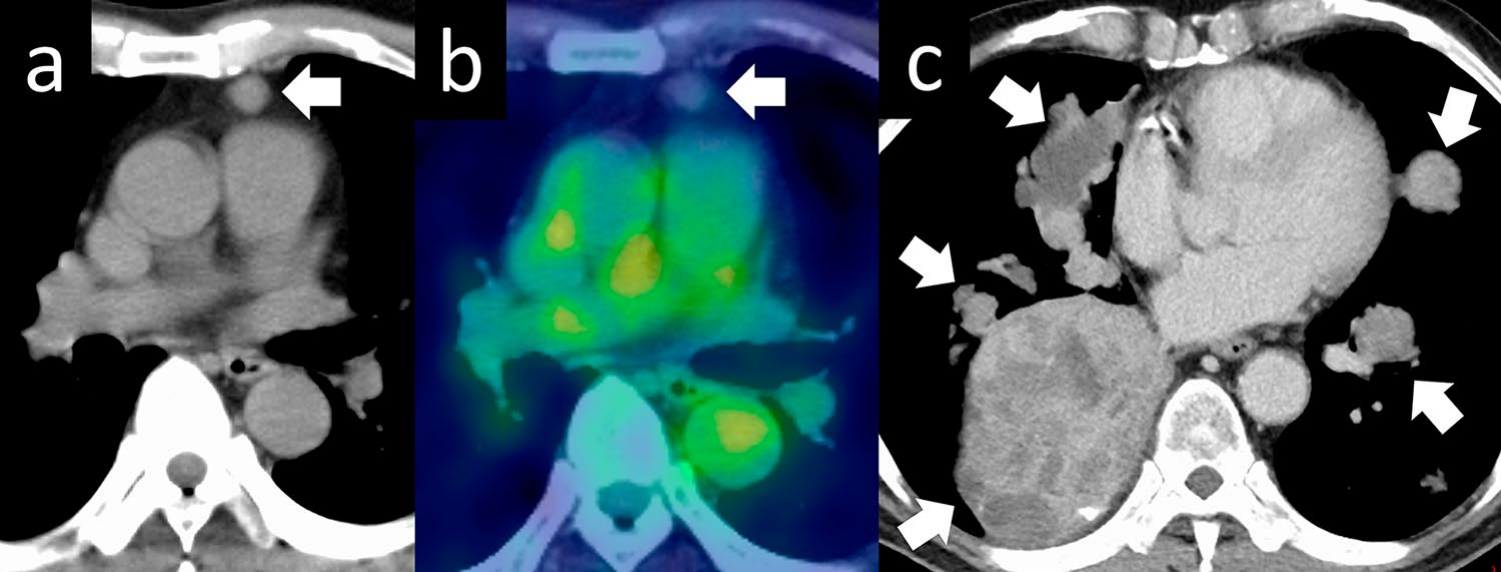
Atypical type A thymoma. A 64-year-old man with an atypical subtype of type A thymoma. a Chest CT shows a small round nodule in the anterior mediastinum that did not invade the surrounding organs (arrow). b 18F-FDG PET shows no hypermetabolism in the nodule (arrow). c CT scan is taken 10 years after surgery. The patient relapses and has multiple lung, bone, and brain metastases (arrows). CT, computed tomography; 18F-FDG PET, 18F-fluorodeoxyglucose positron emission tomography
